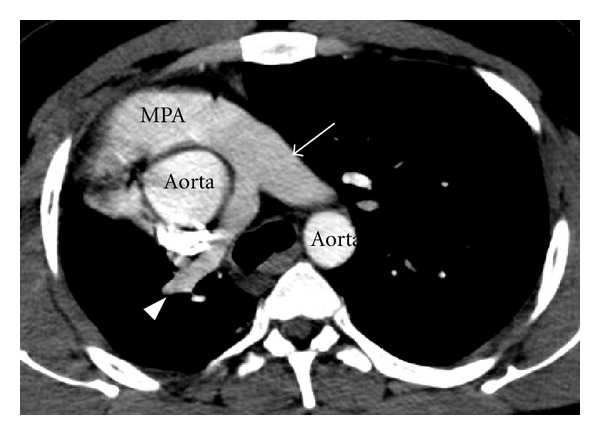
CT angiography axial image depicts smaller calibre right pulmonary artery (arrow head), normal calibre left pulmonary artery (thin arrow), main pulmonary artery (MPA).
